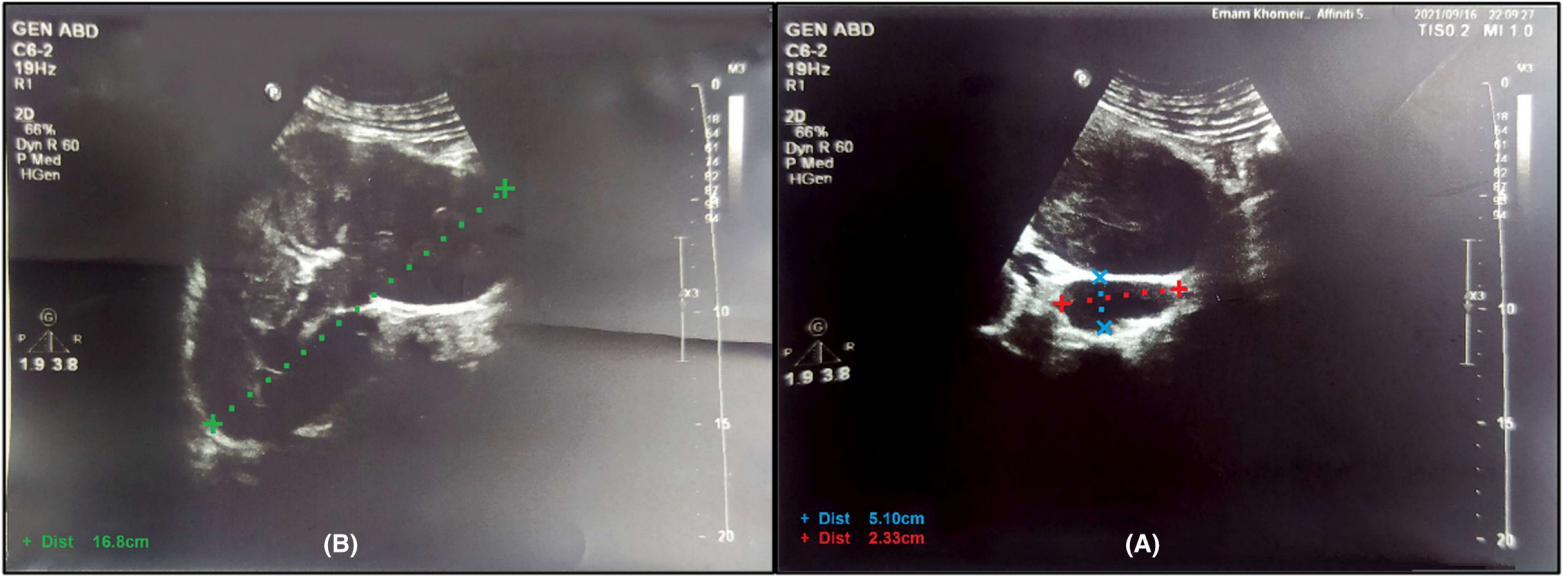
Color Doppler ultrasound of the left kidney. (A) Renal vein thrombosis in the left renal hilum measuring 51 × 23 mm (blue and red dotted lines) (B) Left kidney swollen and larger than normal with a longitudinal diameter of 168 mm (green dotted line)
